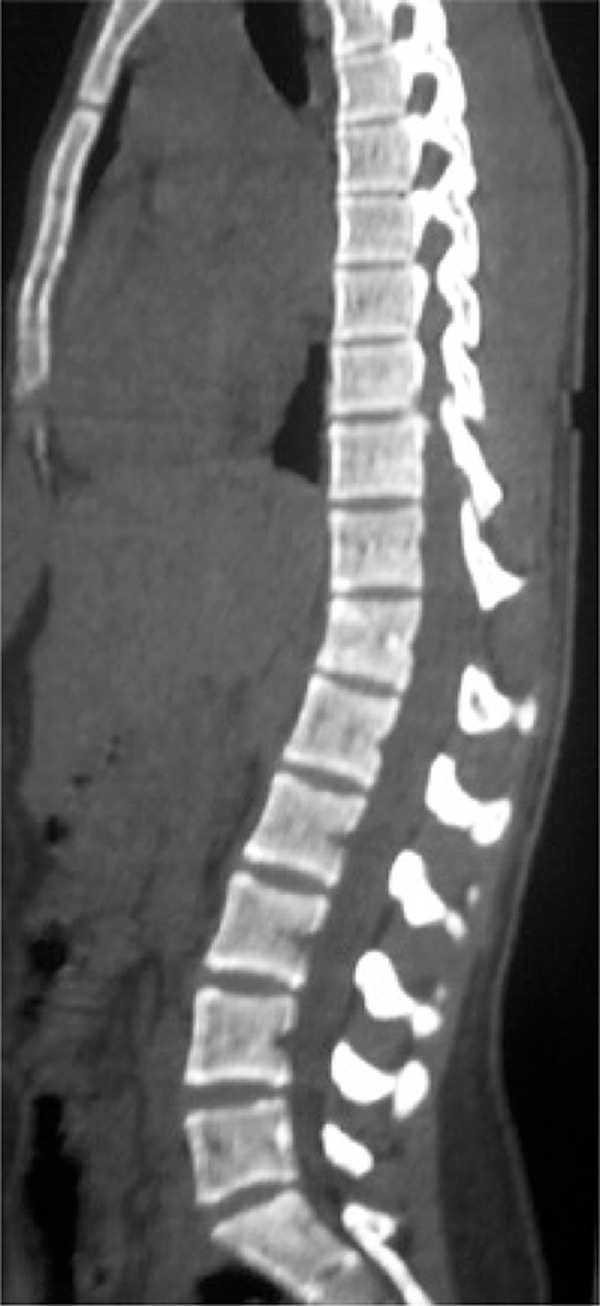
Sagittal computed tomography scan showing an enlargement of T11 and T12 spinous processes, which suggests a ligament injury
